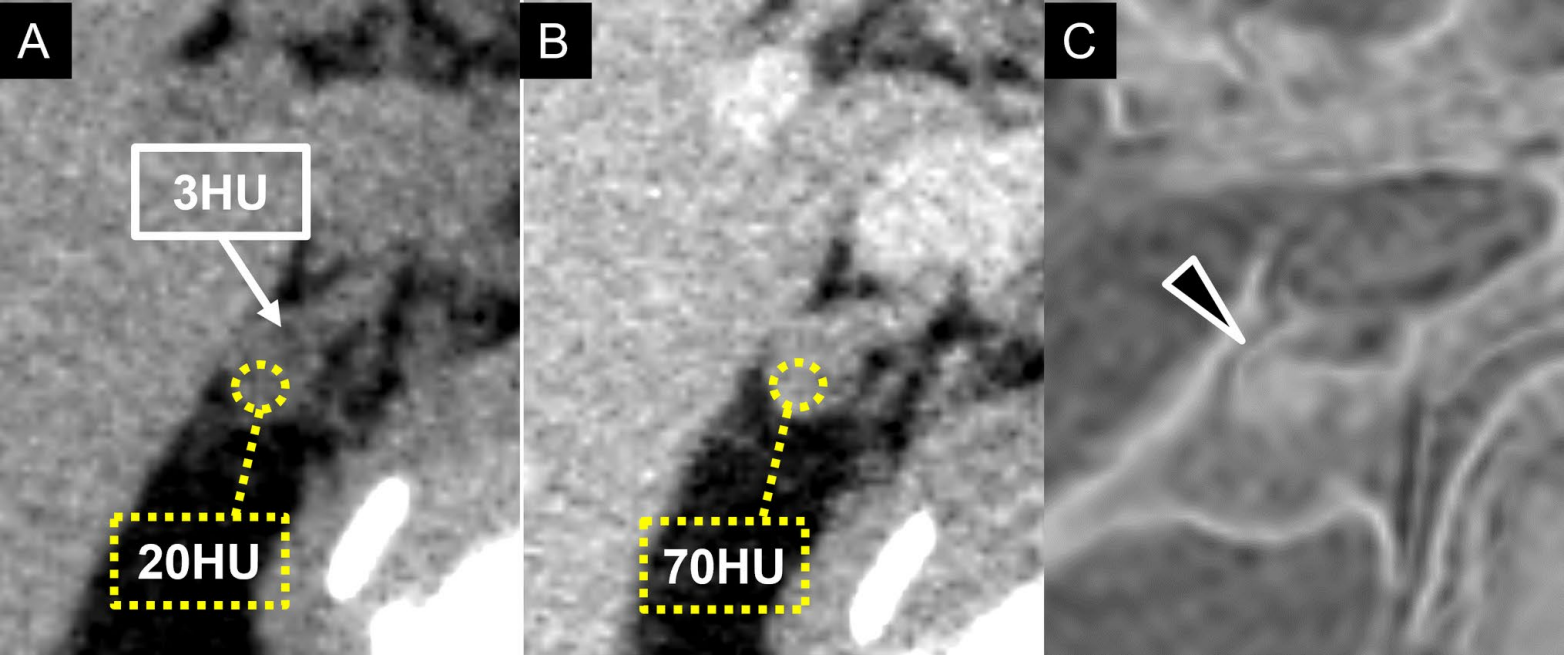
Computed tomography and magnetic resonance imaging findings in an aldosterone-producing adenoma. A 73-year-old male with hypertension and nocturia diagnosed with primary aldosteronism based on an aldosterone–renin ratio > 200 in the captopril challenge test. A Unenhanced CT shows a low-attenuation area (3 HU) anteriorly in the right-adrenal mass (arrow), indicating the presence of fat content. B The posterior portion of the lesion demonstrates increased attenuation from 20 HU on the unenhanced phase to 70 HU on the portal venous phase (with a relative enhancement ratio of 250%), exceeding the cutoff value of 210%, consistent with a cortical adenoma. C A subtraction image of chemical-shift imaging suggests lipid content within the lesion. Adrenal venous sampling confirmed this region as an aldosterone-producing adenoma, and the patient underwent surgical resection for definitive treatment
